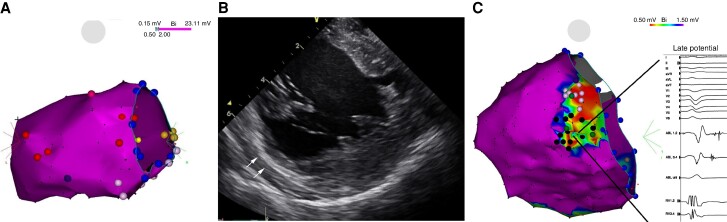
Electroanatomical voltage maps (CARTO 3, Biosense Webster) of the left ventricle in a patient with dilated cardiomyopathy and electrical storm. (*A*) Left panel shows an endocardial map with preserved voltage; (*B*) this panel displays intra-cardiac echocardiography with a clear mid-myocardial scar (arrows); and (*C*) this panel demonstrates an epicardial map with area of low voltage and late potentials in posterolateral region.
